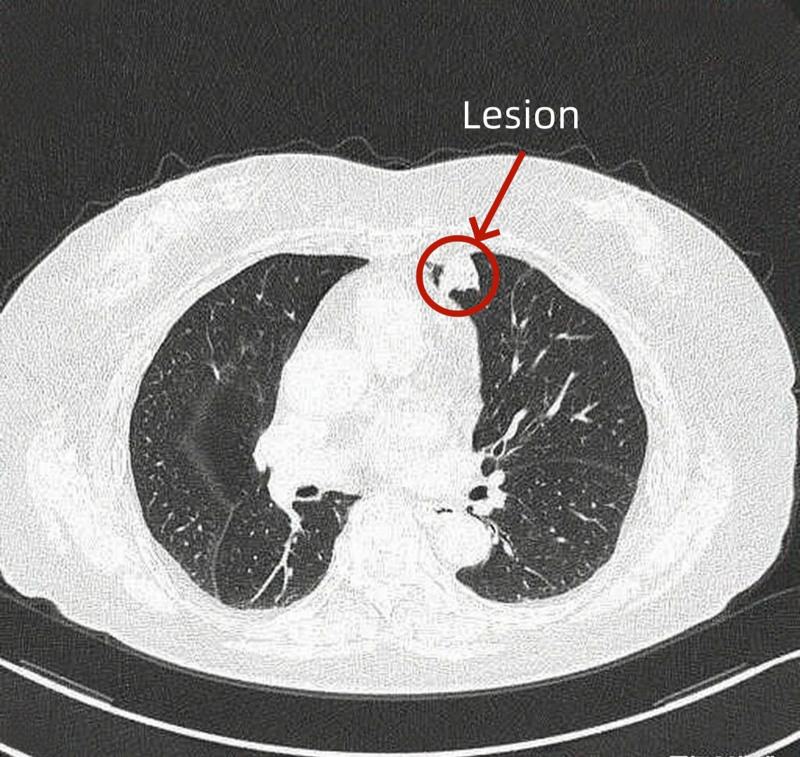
High-resolution computed tomography (HRCT) of the lungs in November 2024.
